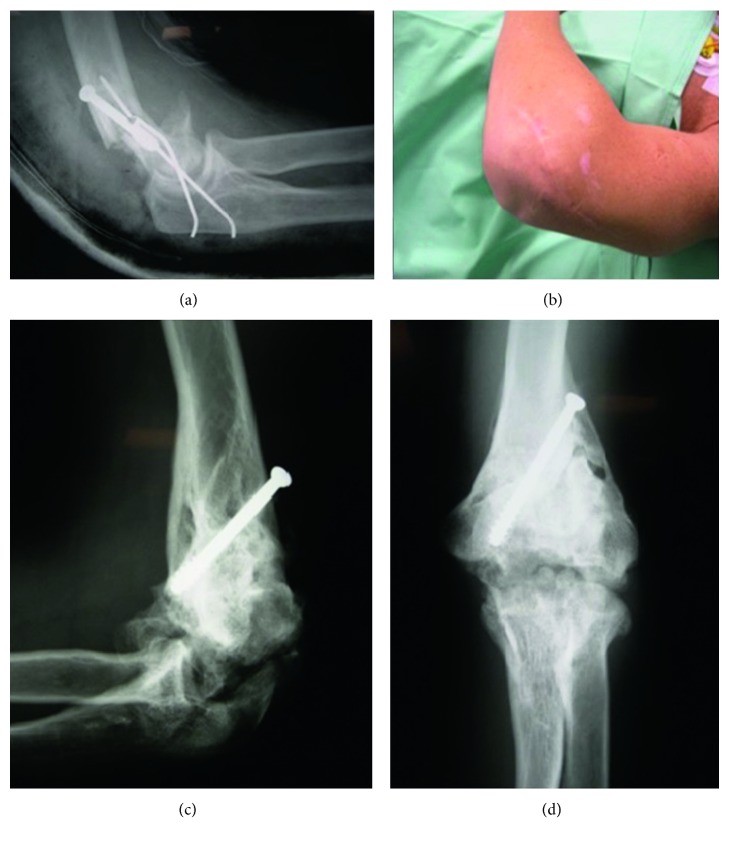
Direct postoperative radiograph showing the insufficient fracture fixation after previous surgery (a); the skin showing the scar following previous surgery (b); radiographs showing distal humeral malunion and generalized elbow joint osteoarthritis (c, d).
